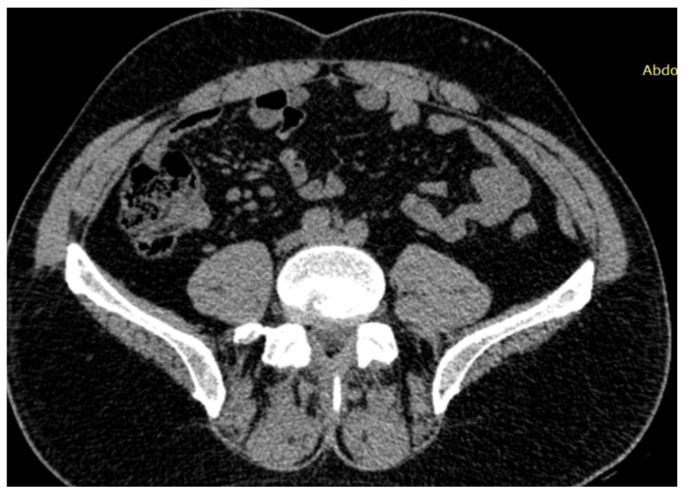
CT showing clustered mesenteric lymph nodes with inflammatory features.
